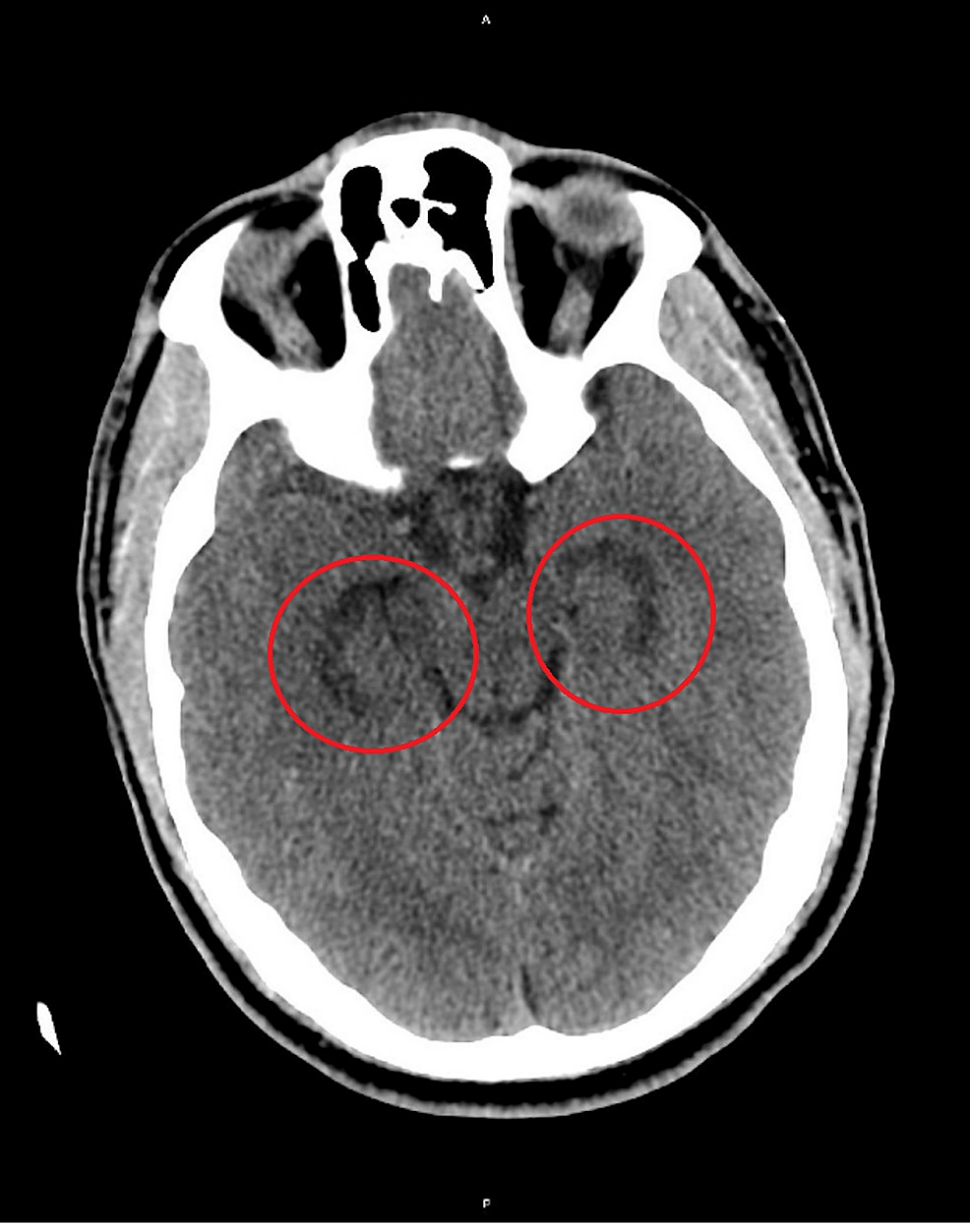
Symmetrical edema in bilateral hippocampi (red circles) in an otherwise unremarkable CT head (axial view) without contrast.
